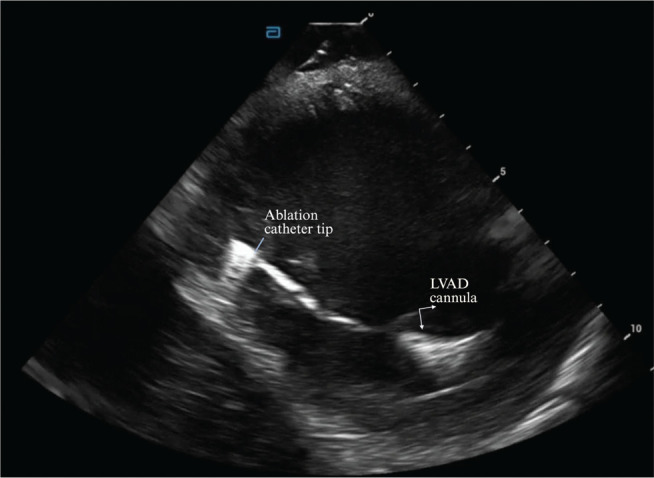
Intracardiac echocardiography image demonstrating the ablation catheter tip positioned adjacent to the left ventricular assist device inflow cannula within the left ventricle. *Abbreviation:* LVAD, left ventricular assist device.
